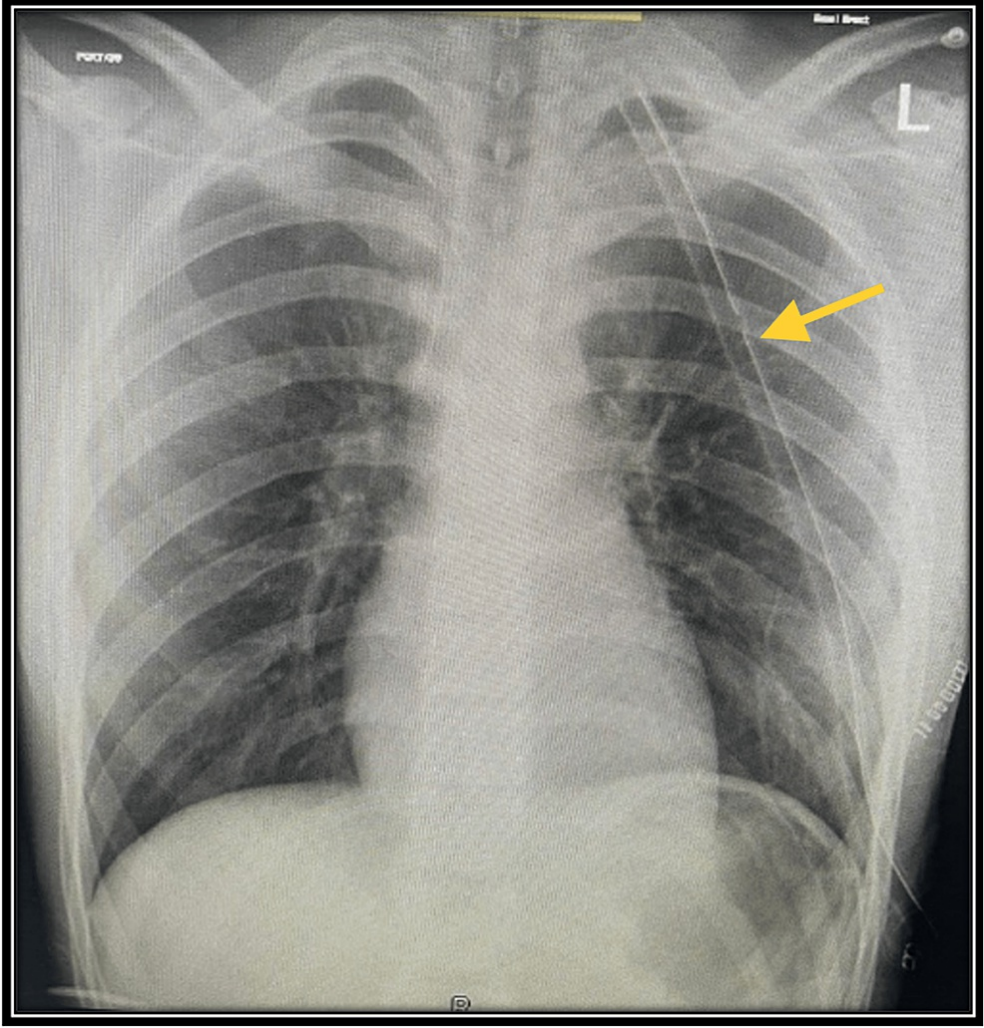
Chest X-ray after surgery showed resolution of hemopneumothorax with fully expanded lung (yellow arrow is the chest tube).
